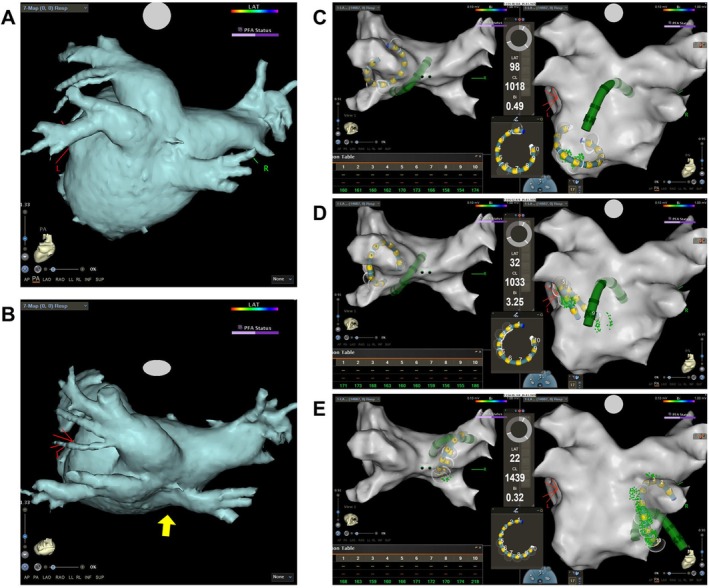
Representative case demonstrating the technique described in this report in an anatomically constrained left atrium. (A, B) Three‐dimensional reconstructed computed tomography images of the left atrium: (A) posterior view and (B) superior view, demonstrating compression of the posterior wall of the left atrium by the vertebra (yellow arrow). The left atrial volume was 47 mL. (C–E) Representative catheter manipulation on three‐dimensional mapping: (C) sheath deflection with the ring deployed and fully expanded; (D) catheter withdrawal resulting in near‐parallel alignment of the Varipulse ring with the sheath shaft; and (E) clockwise sheath rotation achieving stable and flush contact of the ring at the posterior antrum of the right pulmonary veins. The corresponding video is provided as Video S2.
